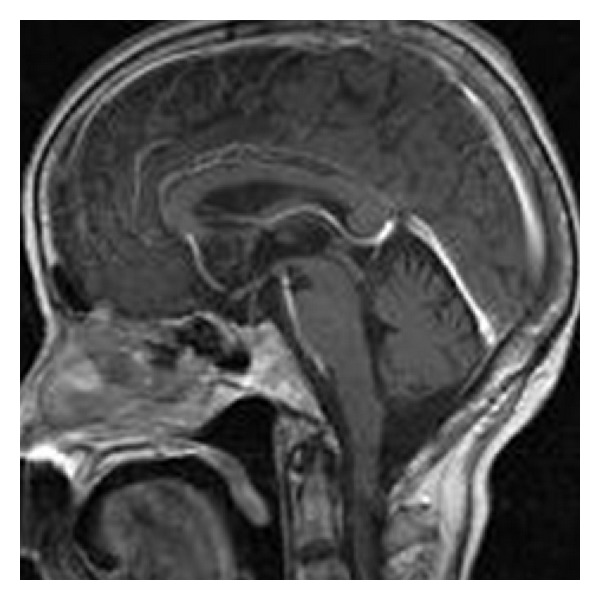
Sagittal Gd-enhanced brain MRI after 5-course chemotherapy shows complete disappearance of the tumor.
